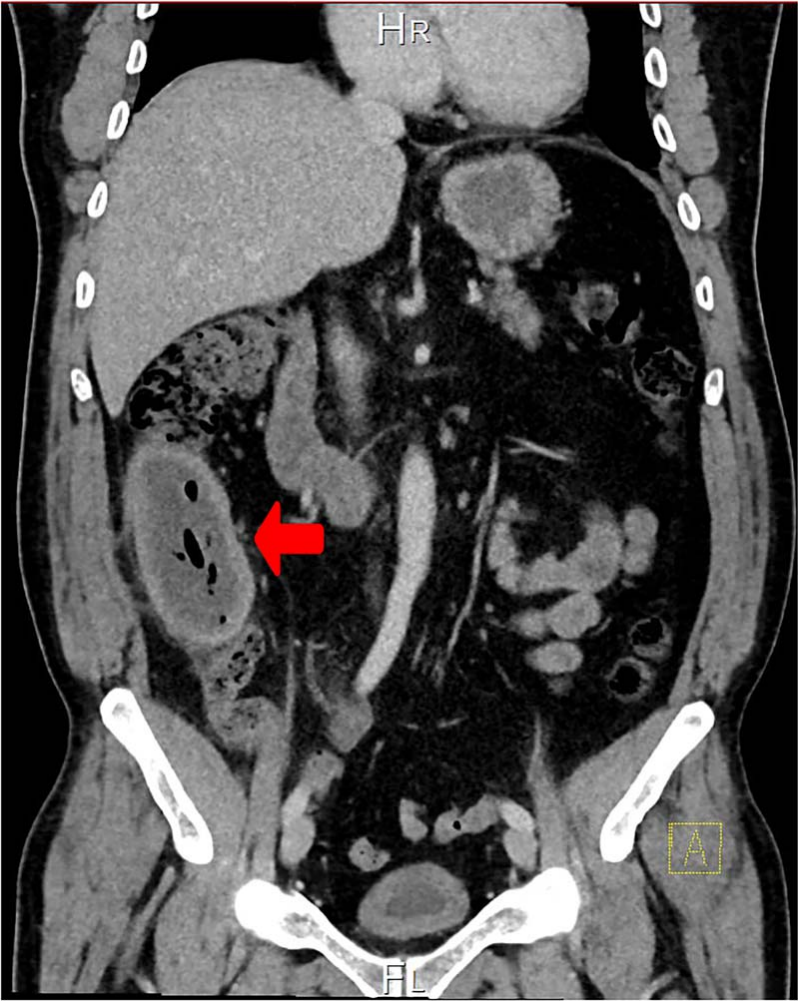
A coronal CT view reveals an oval mass in the right iliac fossa. The mass has a thick, edematous wall and contains fluid and gas. Extensive fat stranding is visible, but no free fluid or bowel dilation is noted.
